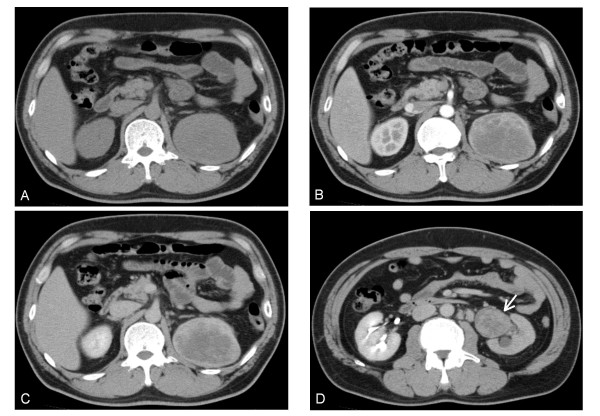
Horizontal section of preoperative abdominal CT. Plain (A), early-phase contrast enhancement (B), and late phase (C) showed contrast enhancement. A tumor thrombus in the left renal pelvis (D, arrow), and its contrast enhancement was similar to the main tumor.
